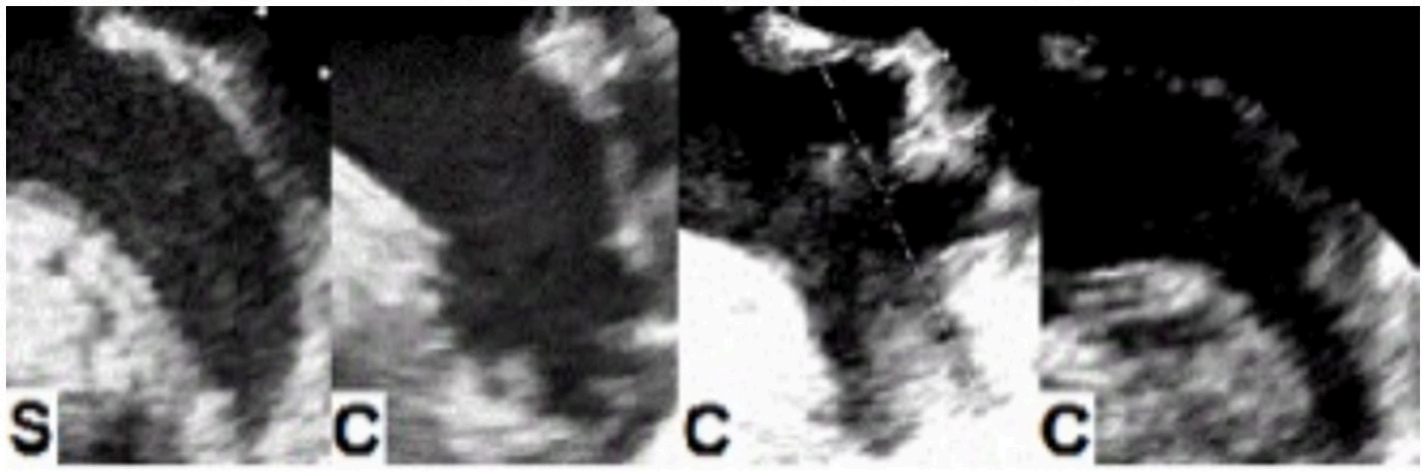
Transesophageal images of left atrial appendage (LAA) morphologies. Functional classification of LAA based on thrombogenicity. Left to right: S, simple LAA or SLA2M (chicken wing based on prior classifications); C, Complex LAA or CLA2M (includes chicken wing with pectinated walls and other complex morphologies including cauliflower, cactus and windsock).
